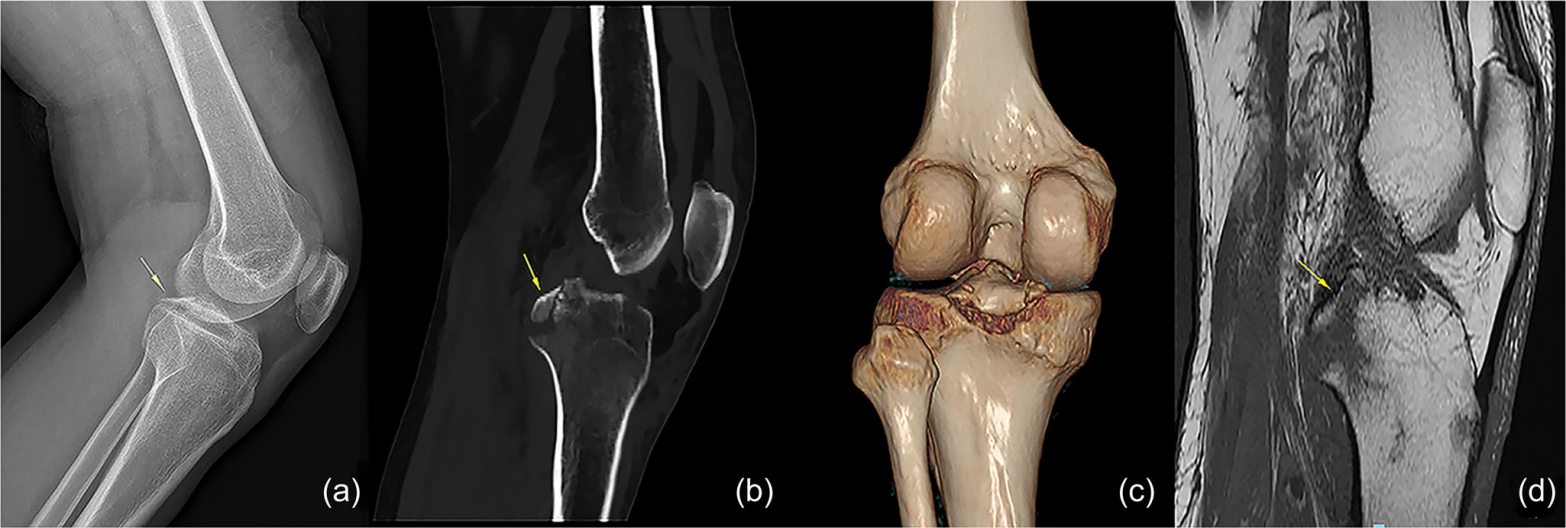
Preoperative imaging of PCL tibial avulsion fractures. (a) Knee x‐ray, (b) sagittal section scan and (c) 3D CT scan reconstruction showed slightly proximally displaced avulsion fracture of PCL. (d) MRI demonstrated loose PCL with tibial attachment slightly displaced proximally. The yellow arrows show the avulsion fragment. 3D, three‐dimensional; CT, computed tomography; MRI, magnetic resonance imaging; PCL, posterior cruciate ligament.
